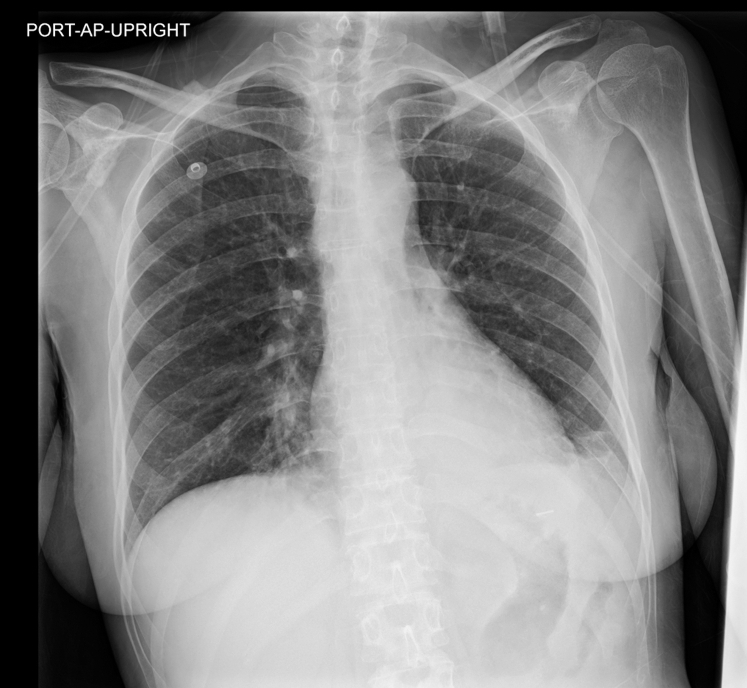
Upright anteroposterior chest radiograph demonstrating a medial opacity at the right lung base with larger consolidation in the retrocardiac region.
